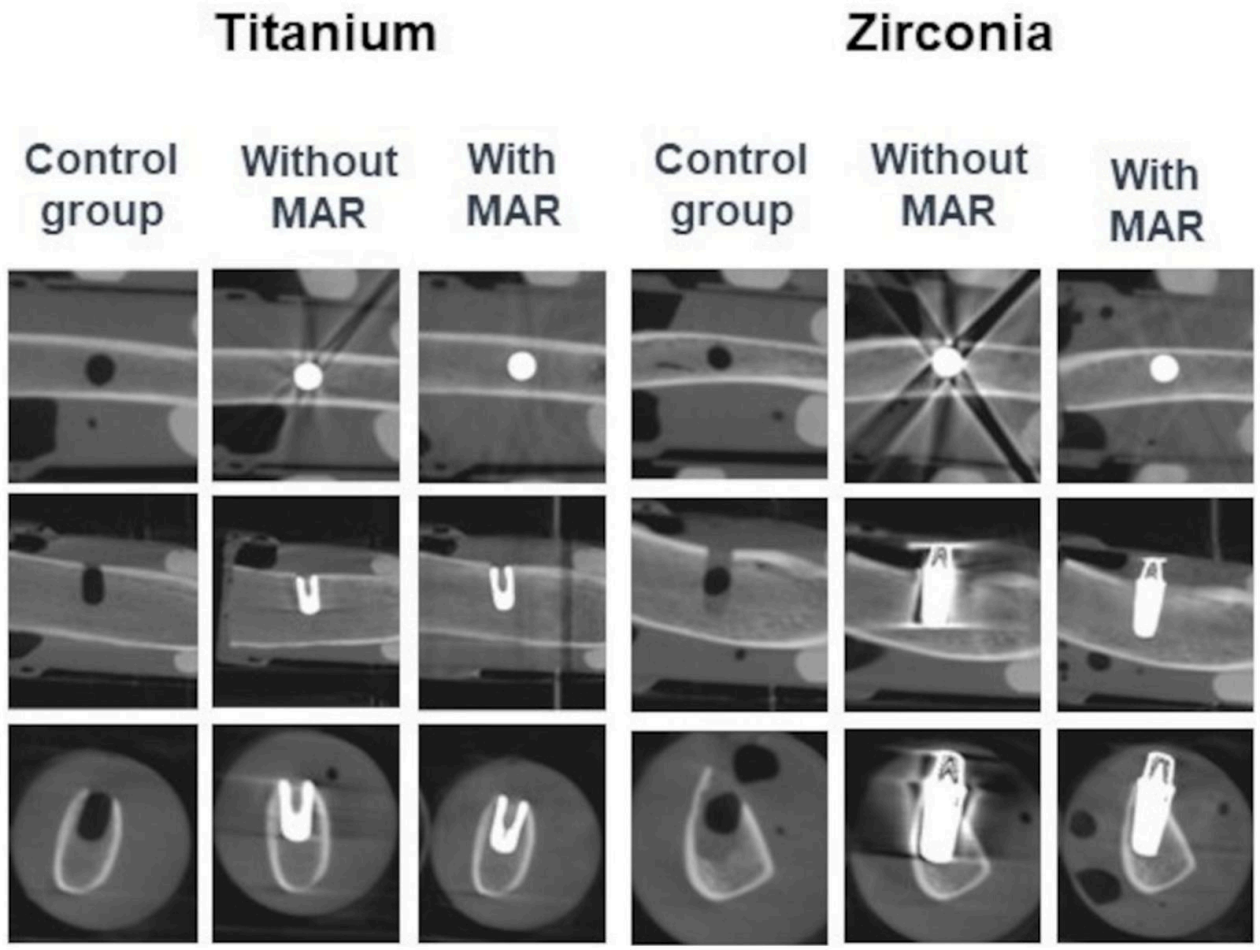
Artefacts caused by titanium and zirconia dental implants in CBCT images acquired according to the use of metal artefact reduction (MAR).
